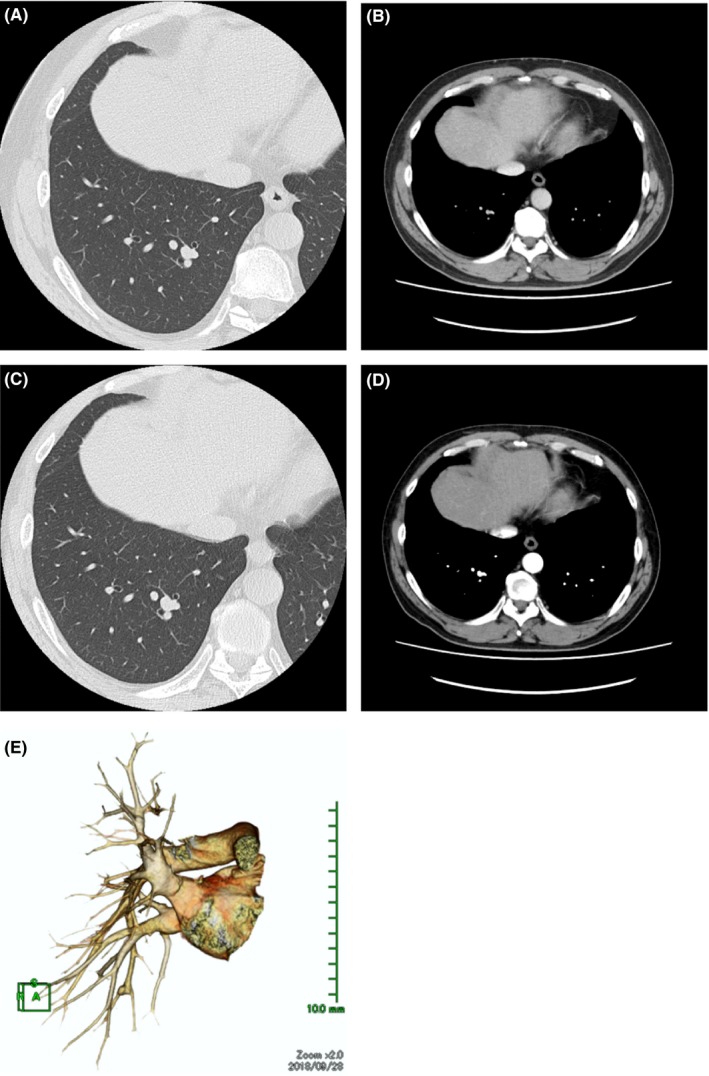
A, High‐resolution computed tomography (CT) scan image showing an 8.6 mm diameter aneurysm in the periphery of right pulmonary artery 10. B, Contrast‐enhanced CT scan images showing an 8.6 mm diameter aneurysm in the periphery of right pulmonary artery 10. C, The aneurysm's diameter has increased to 9.9 mm one year later. D, The aneurysm's diameter has increased to 9.9 mm one year later. E, Three‐dimensional reconstruction of contrast‐enhanced CT scan showing the aneurysm at the branch of A10b and A10c
